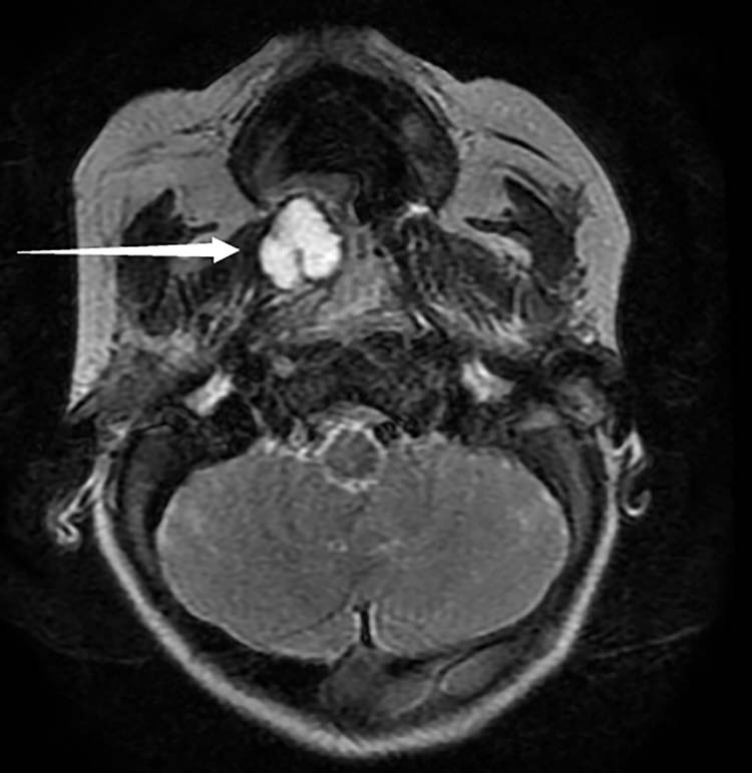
Pictured is an MRI displaying a 1.6 × 1.4 × 1.5-cm cystic submucosal mass arising from the right soft palate.
